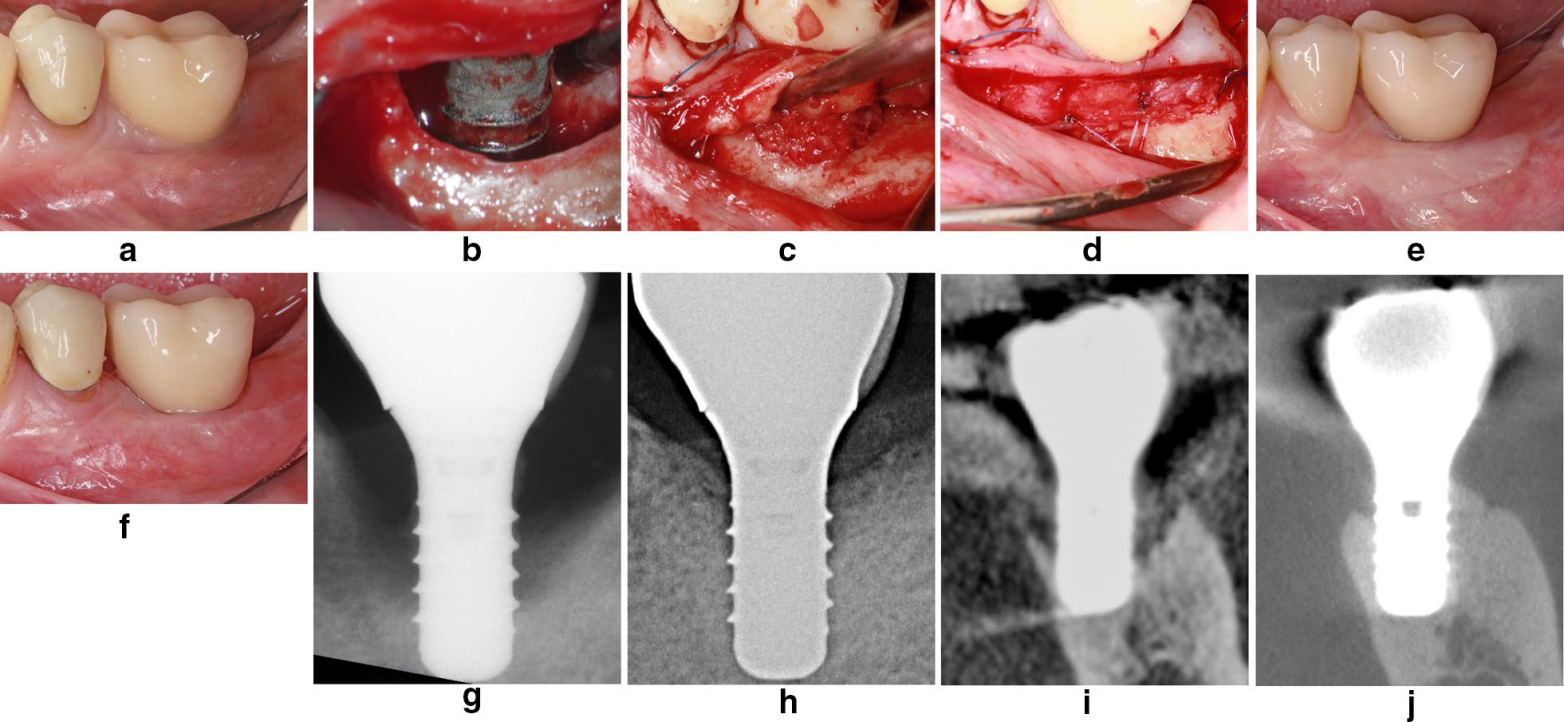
a Severe peri-implantitis lesion with suppuration and an insufficient width of the keratinized mucosa. b Implant surface decontamination with the Er:YAG laser. c Hard and soft tissue grafting according to the LAPIDER technique. d Periosteal suturing to cover the autogenous grafts. e Uneventful healing 9 months after LAPIDER surgery, but still without a sufficient zone of attached mucosa. f 3-year follow-up examination after an additional apically positioned flap with FGG. g Severe interproximal bone loss at pre-operative examination. h Radiograph at 3-year follow-up examination reveals significant vertical bone regeneration. i Severe buccal and lingual bone loss at baseline. j CB-CT at 3-year follow-up examination shows a pronounced buccal and lingual bone regeneration
